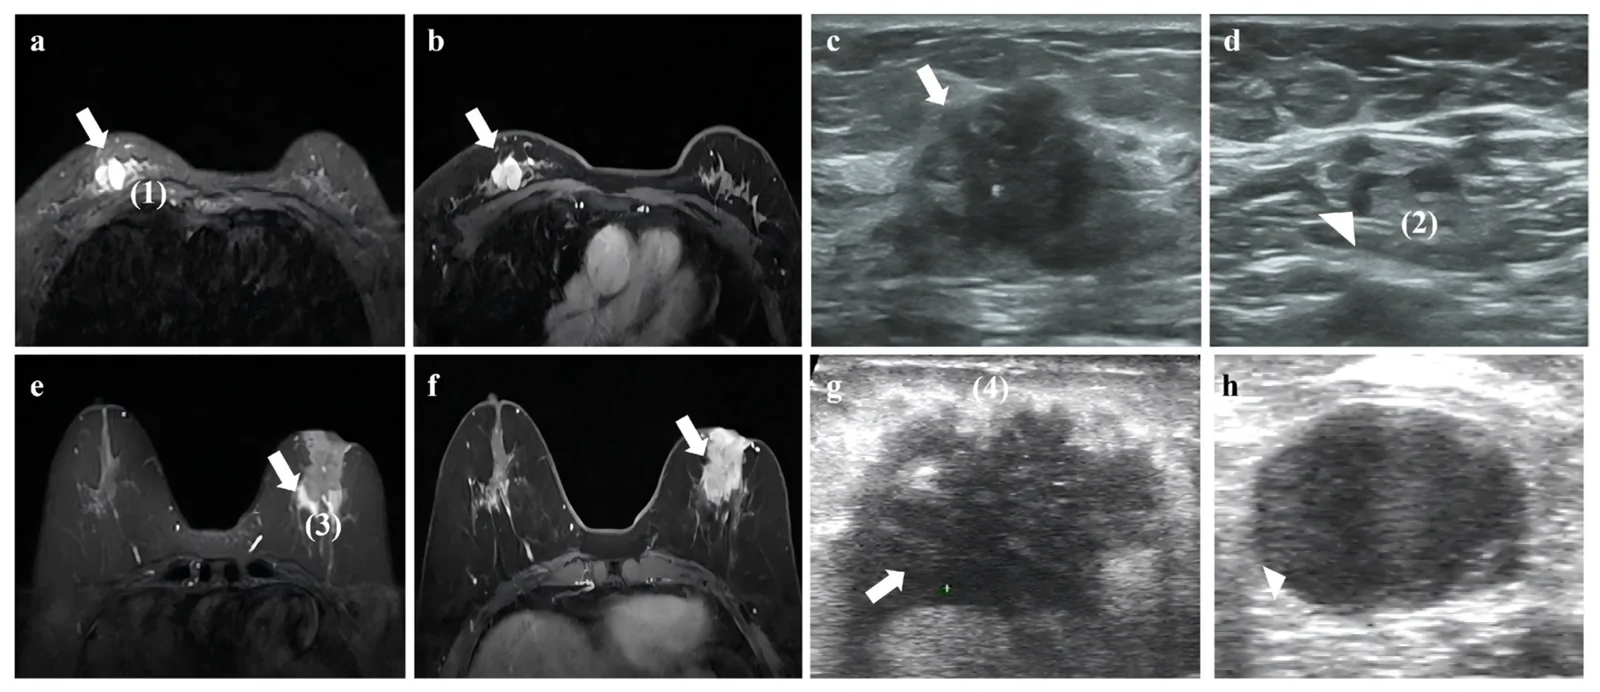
Examples of pretreatment MRI and US in two cN+ breast cancer patients. (a–d) A 59-year-old woman (cT2, HER2-positive subtype, Ki-67 15%) achieved ypN0 after NAC (model: ypN0). (a) T2WI shows the primary tumor with intratumoral T2 hyperintensity (1); T2 signal intensity, hyperintense. (b) DCE-MRI demonstrates lesion enhancement. (c) US shows a parallel-oriented hypoechoic mass. (d) Axillary US shows the target lymph node with a preserved fatty hilum (2); hilum, present. (e–h) A 69-year-old woman (cT2, HR+/HER2− subtype, Ki-67 30%) had residual nodal disease (ypN1–3) after NAC (model: ypN1–3). (e) T2WI shows peritumoral edema adjacent to the primary tumor (3); peritumoral edema, present. (f) DCE-MRI demonstrates lesion enhancement. (g) US shows an echogenic rind (4); echogenic rind, present. (h) Axillary US shows the target lymph node. Arrows indicate the primary tumor and arrowheads indicate the target lymph node; (1)–(4) denote the corresponding imaging descriptors.
